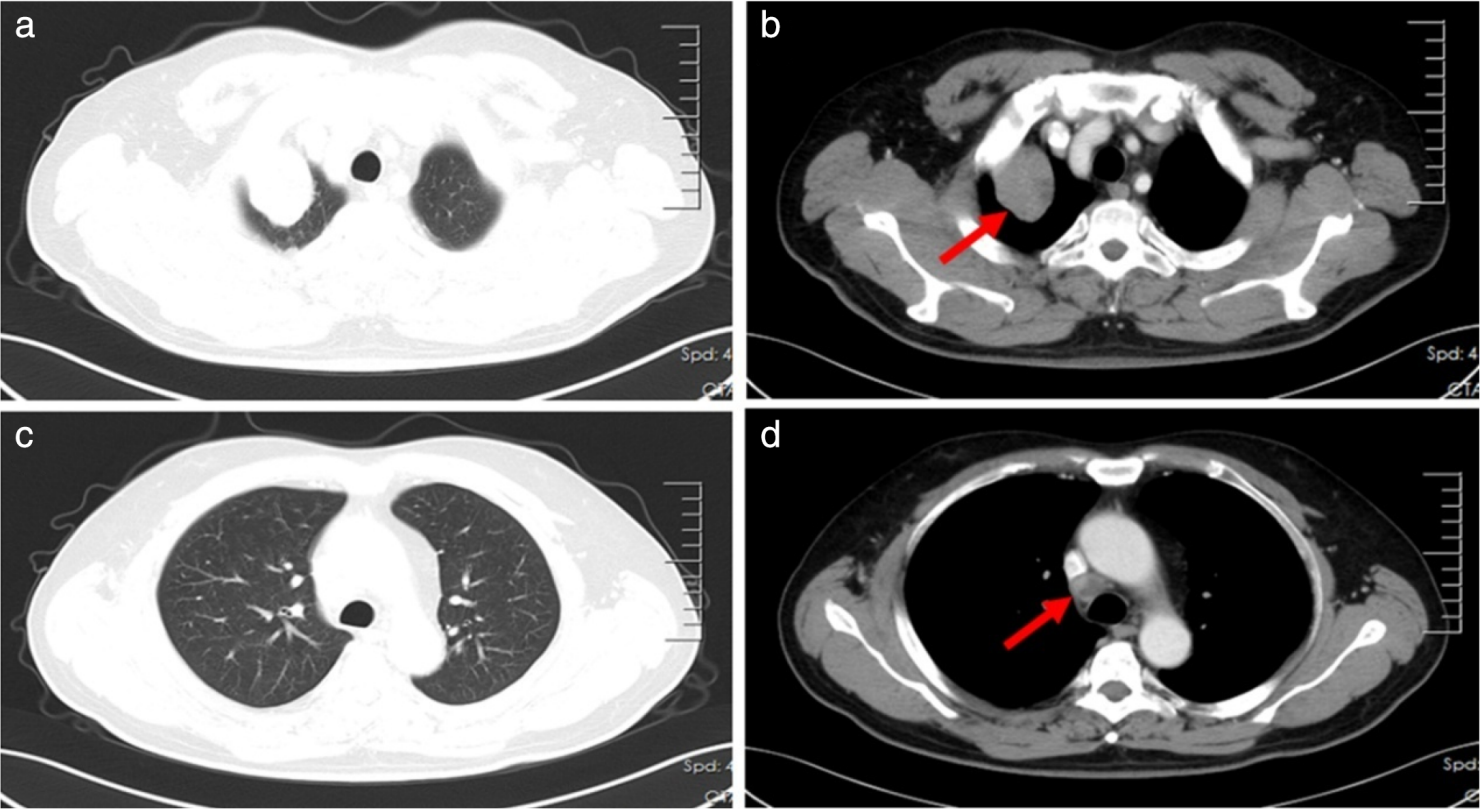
Chest CT images showed a mass in the apical segment of the right upper lung and enlarged mediastinal lymph nodes
